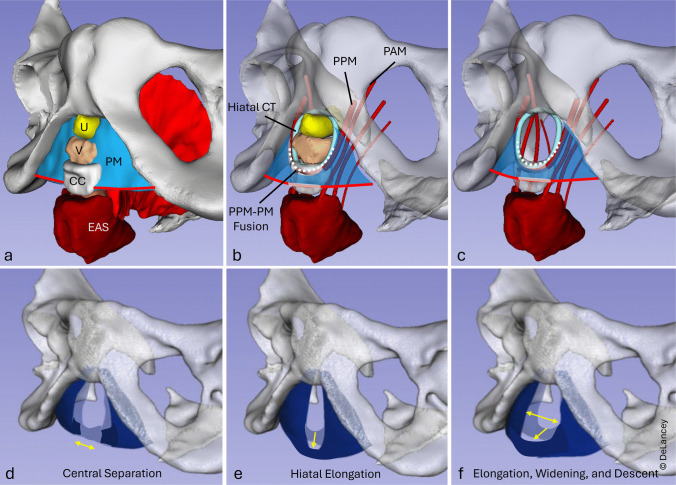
3D model of the perineal complex. Model of the perineal complex from the MRI of a nullipara with normal support (a-c). Teal ring, hiatal connective tissue (Hiatal CT); white dashed line, perineal membrane (PM) and levator ani fusion. (CC, central connection; EAS, external anal sphincter; U, urethra; V, vagina; Levator ani subdivisions: PPM, puboperineal; PAM, puboanal). Bottom row demonstrates examples of perineal membrane changes. Light blue perineal membrane, normal configuration; dark blue, distortion
